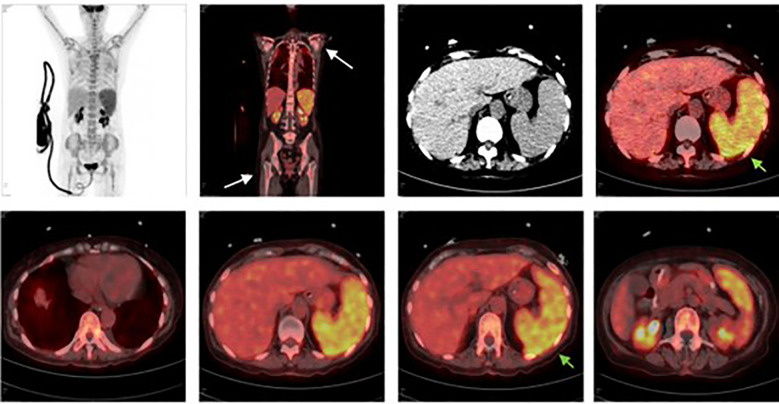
Positron Emission Tomography-Computed Tomography Findings PET/CT demonstrated splenomegaly with diffusely increased FDG uptake (SUVmax 4.74) and mild diffuse hypermetabolism in the bone marrow (SUVmax 4.06). Diffuse increased marrow uptake was also observed in the bilateral patellae, femora, and tibia-fibulae, with the most prominent lesion located in the left patella (SUVmax 5.31). These findings raised suspicion for occult hematologic malignancy and supported repeat bone marrow evaluation.
